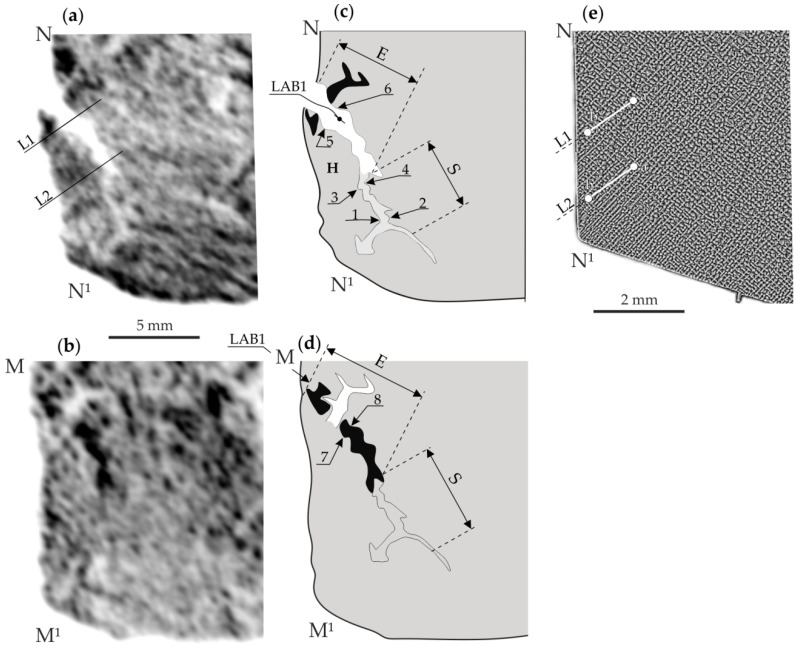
Examples of X-ray topograms obtained from fragment A of surface S_1_ and fragment A* of the S_2_ surface (a,b) with their schemes (c,d) and the dendritic structure visualised on surface S_1_ (e). Since the topogram from fragment A* was obtained from the mirror surface of fragment A, it was transformed by mirroring for easier comparison of areas of the same LAB visualised on both surfaces S_1_ and S_2_.
